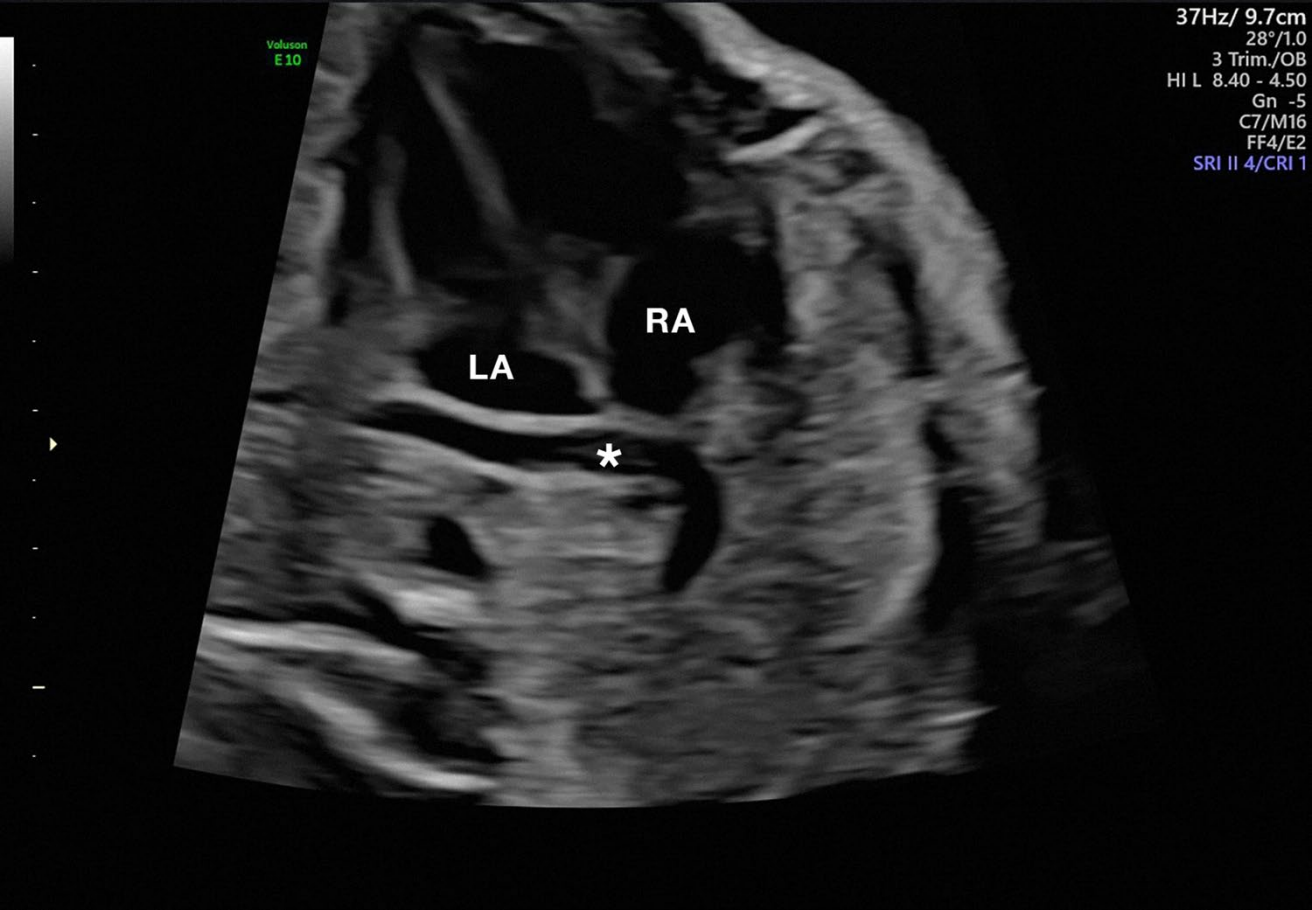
Ultrasound image of supracardiac TAPVC: the pulmonary veins connect to a common confluent vein behind the heart (*), draining via a vertical vein to the brachiocephalic vein and superior vena cava. *RA* right atrium; *LA* left atrium
